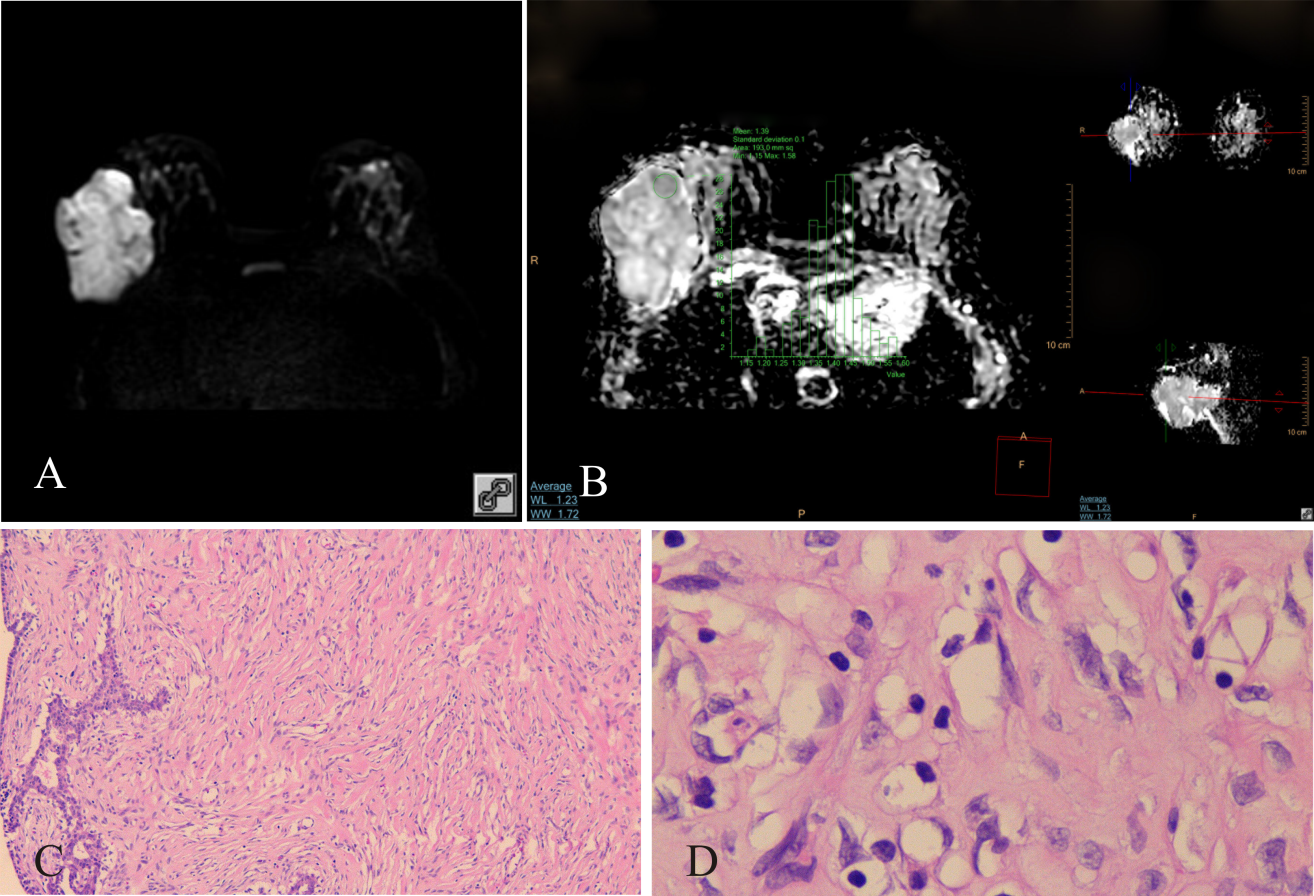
Benign PT of the right breast, female, age 46 years. (A) DWI (b800s/mm^2^): mixed hyperintense signal; (B) ADC map: isointense–hyperintense mixed signal, ADCmean = 1.39 × 10^−3^ mm^2^/s; (C) HE ×100: tumor stromal cells were dispersed; (D) HE ×400: no nuclear division was observed, tumor cells were loosely arranged.
